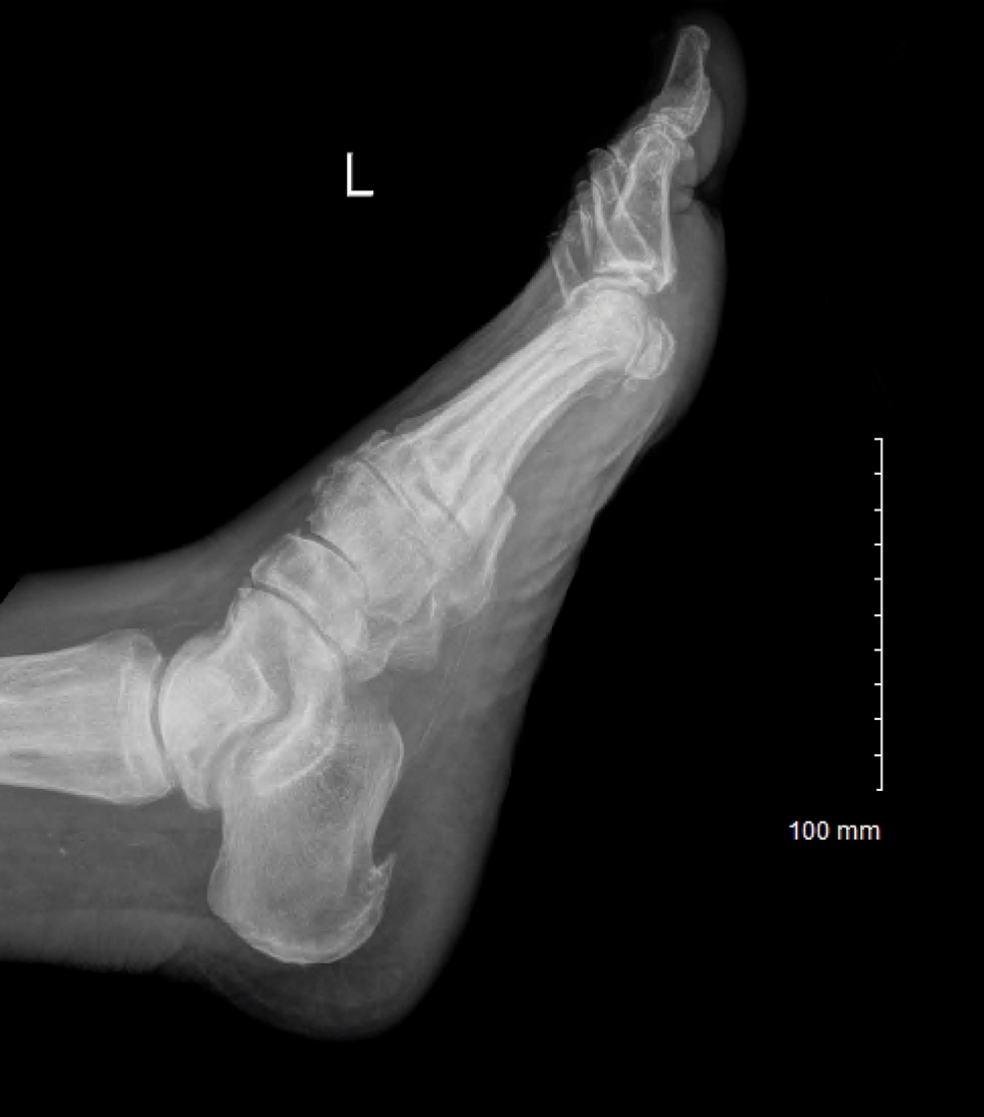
Lateral view X-ray of the left foot.
